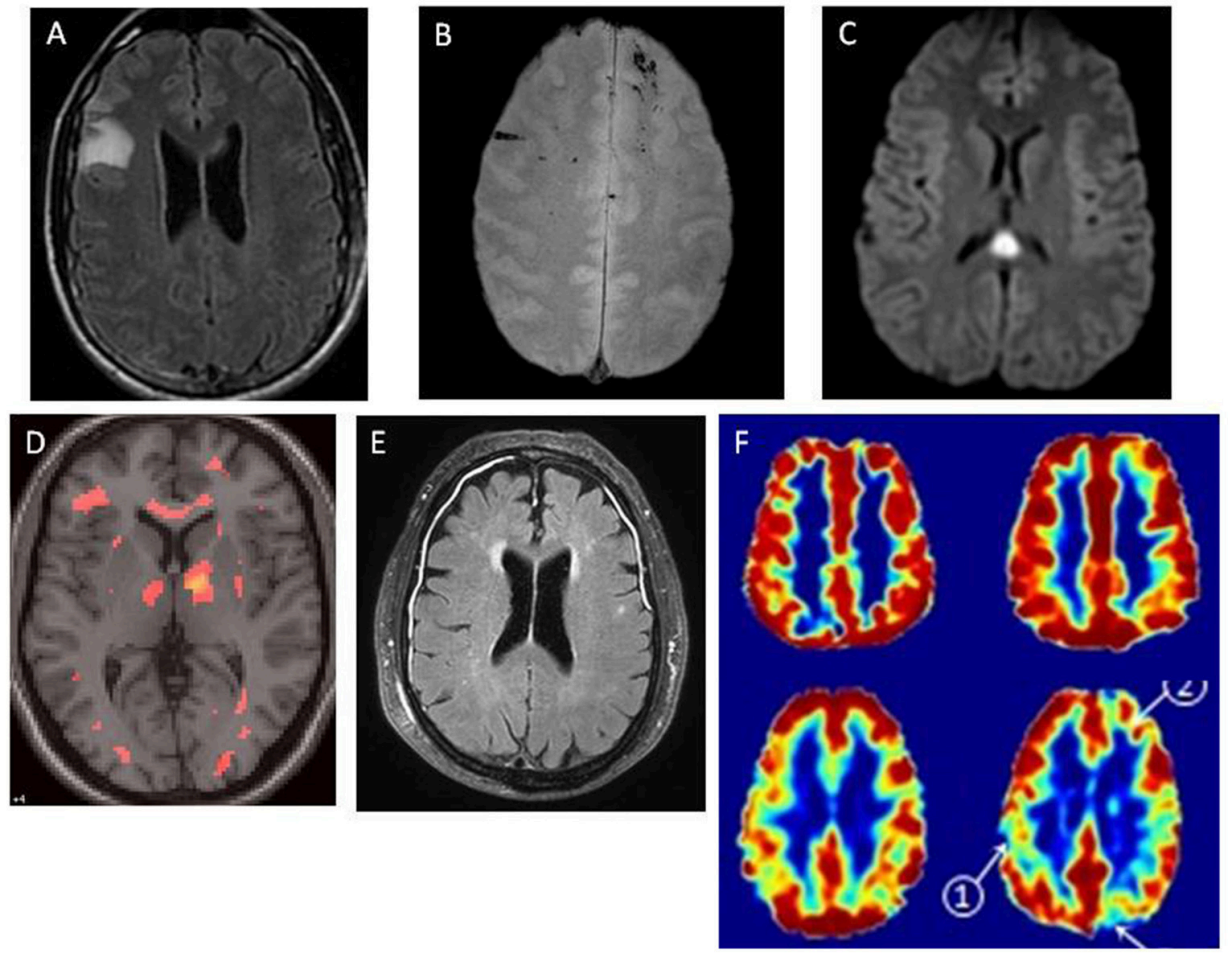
Magnetic resonance images of patients with post-concussion symptoms. MRI findings in patients with mTBI, demonstrating multiple pathologies. In each case, cranial CT was normal. MRI was obtained within 48 h on injury. (A) Right frontal non-hemorrhagic contusion, noted on FLAIR image. (B) Linear microhemorrhages in left and right frontal lobes, noted on T2^*^ image. (C) Diffuse axonal injury lesion in splenium of corpus callosum, with restricted diffusion noted on DWI image. (D) Diffuse axonal injury, with multifocal lesions noted on diffusion tensor imaging (DTI). (E) Traumatic meningeal enhancement of subdural effusions, noted on post-gadolinium FLAIR image. (F) Traumatic microvascular injury. - Top row represents a single healthy control. Bottom row represents a single TBI patient.- Left column: Cerebral Blood Flow (CBF), assessed by arterial spin labeling.- Right column: Cerebrovascular reactivity (CVR) assessed using BOLD response to hypercapnia. Credit for figures: Figures A, B, C, E: Larry Latour, PhD, NINDS/NIH; D: Carlos Marquez de la Plata, PhD, University of Texas at Dallas; F: Franck Amyot, PhD, Uniformed Services University of the Health Sciences.
